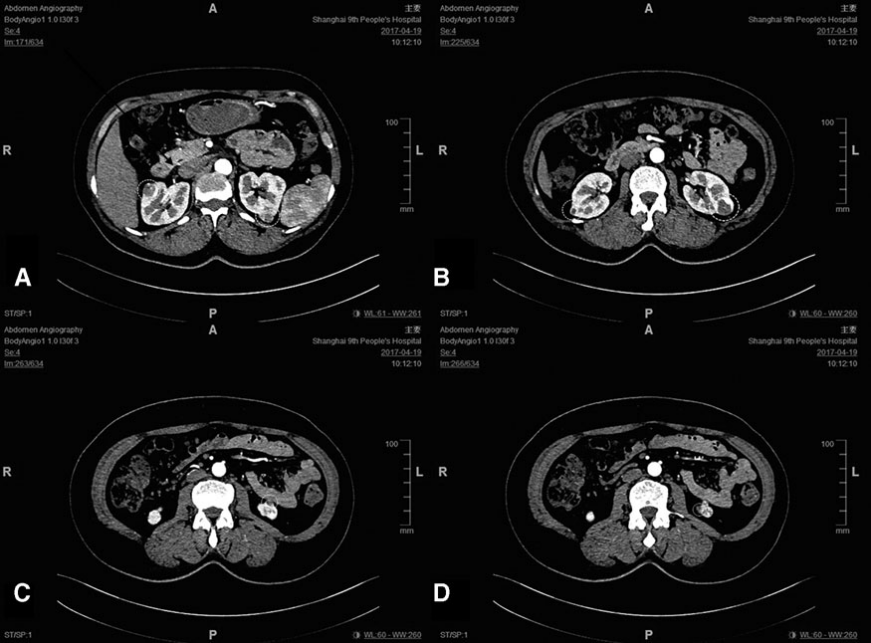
Preoperative contrast-enhanced CT scan of the kidney. (A) A mass in the right kidney and a mass in the upper pole of the left kidney. (B) A mass in the right kidney and a mass in the middle pole of the left kidney. (C) One mass in the lower pole of the left kidney. (D) Another mass in the lower pole of the left kidney. The *dotted circles* indicate the tumor before surgery.
